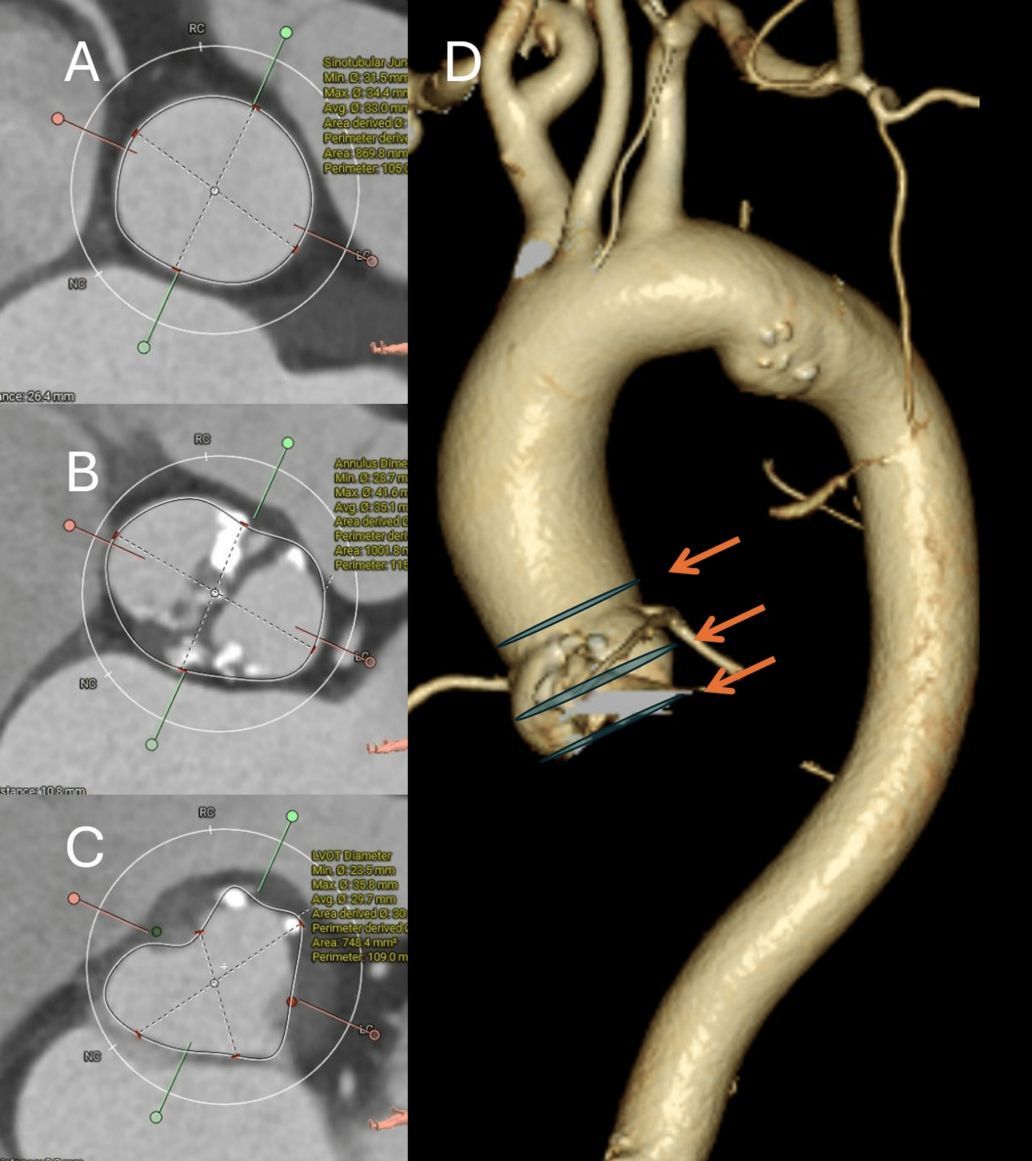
Preoperative computed tomography (CT) images. (A) Cross-sectional view at the sinotubular junction (STJ). (B) Cross-sectional view at the sinuses of Valsalva (SOVs). (C) Cross-sectional view at the left ventricular outflow tract (LVOT). (D) Three-dimensional reconstructed CT image of the aorta. The measured diameters were as follows: Ascending aorta 40×37 mm, STJ 39×29 mm, SOVs 41×28 mm, annulus 39×27 mm, LVOT 41×25 mm, femoral artery (FA) and external iliac artery (EIA) both < 9 mm.
